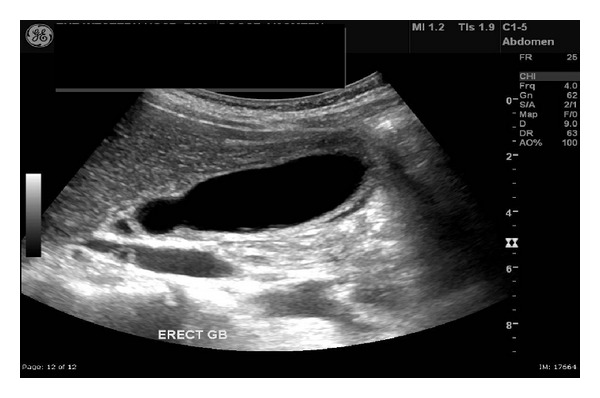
Gallbladder ultrasound showing a distended gallbladder, some pericholecystic fluid, and no gallstones.
